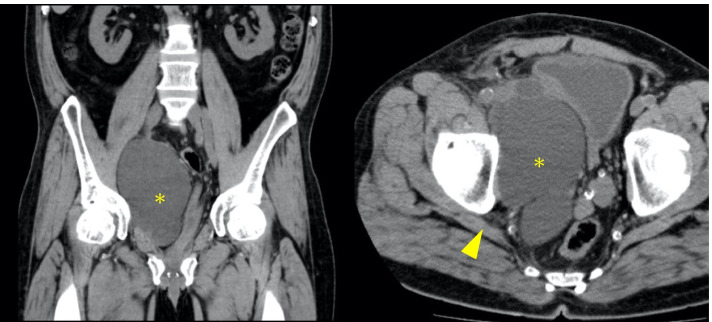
Computed tomography of the pelvis. The coronal scan shows a huge lymphocele with low signal intensity (*). The axial scan shows that a lymphocele (*) compresses the piriformis muscle (arrow)
